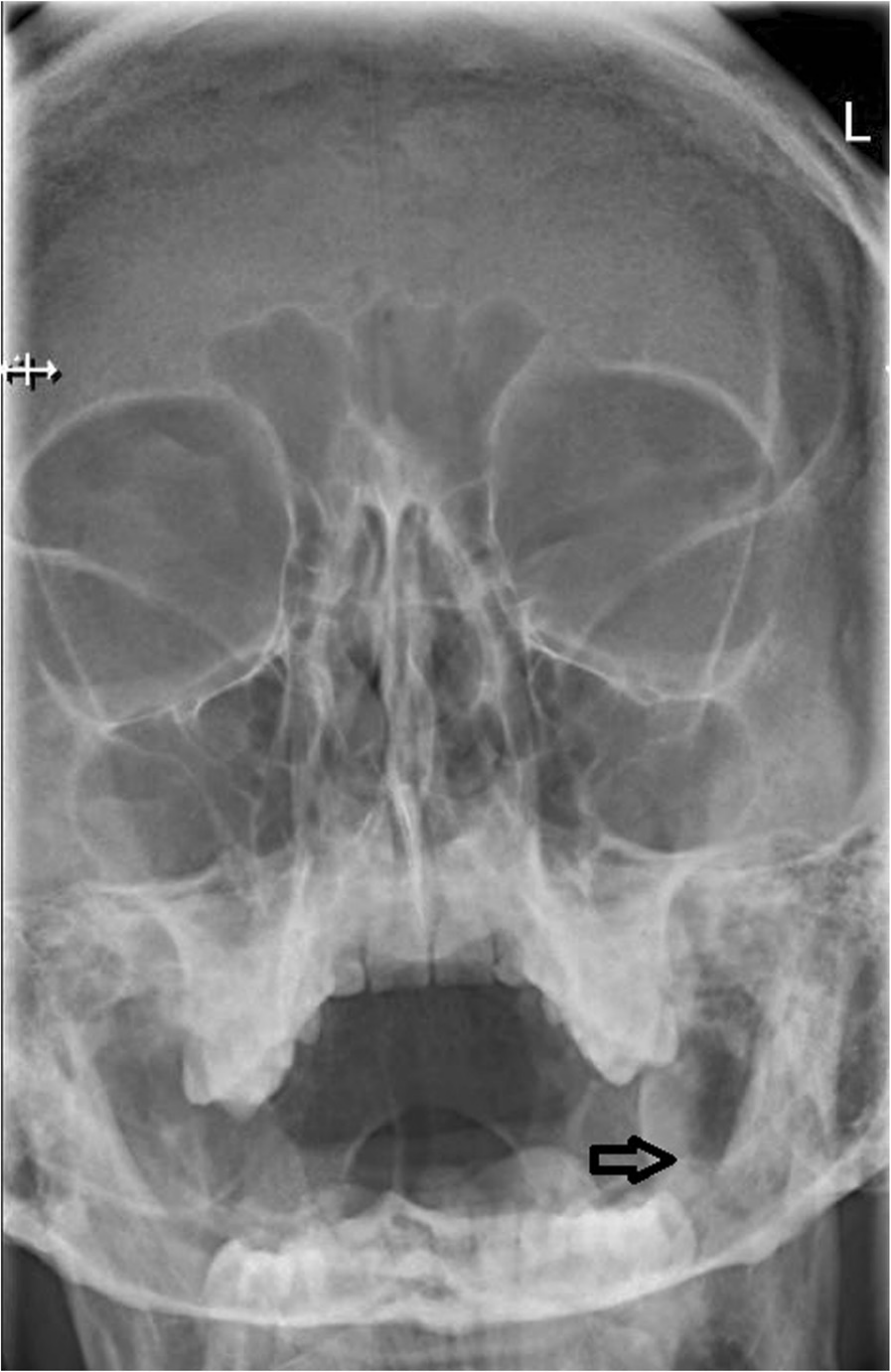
A plain sinus x-ray was taken at the time of patient’s arrival at the emergency room. Based on the radiological report, there were no pathological findings. Due to the projection of pneumatized mastoid processes, the plain sinus x-ray has low sensitivity in detecting subcutaneous emphysema in the facial area.
